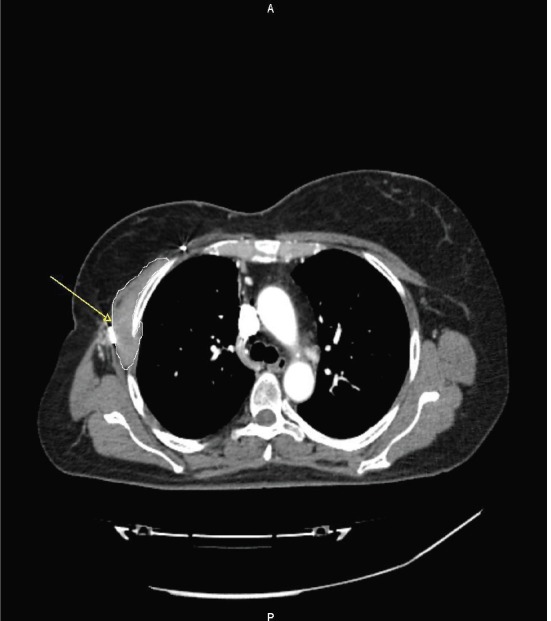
An axial CT scan at recurrence, showing a contrast-enhanced solid tissue (arrow) along the right chest wall, infiltrating the intercostal muscles and the pectoralis muscles, demonstrated at biopsy as recurrence of AF.
